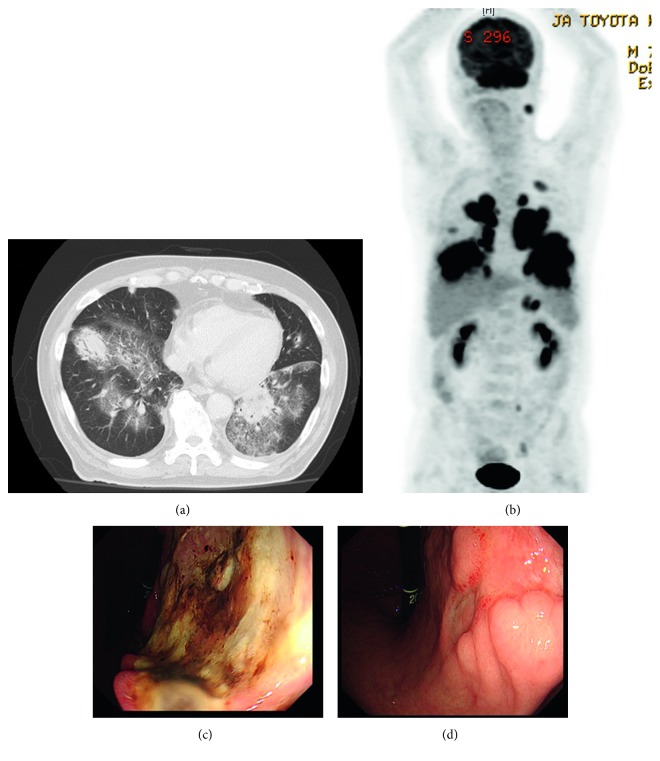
(a) CT of both lungs detects multifocal nodular lesions with ground-glass shadows. (b) PET detects abnormal uptake in multifocal lung lesions and the gastric body. (c) Before treatment, gastroscopy reveals an ulcerative lesion in the gastric body. (d) After treatment, gastroscopy shows improvement of the gastric lesion.
